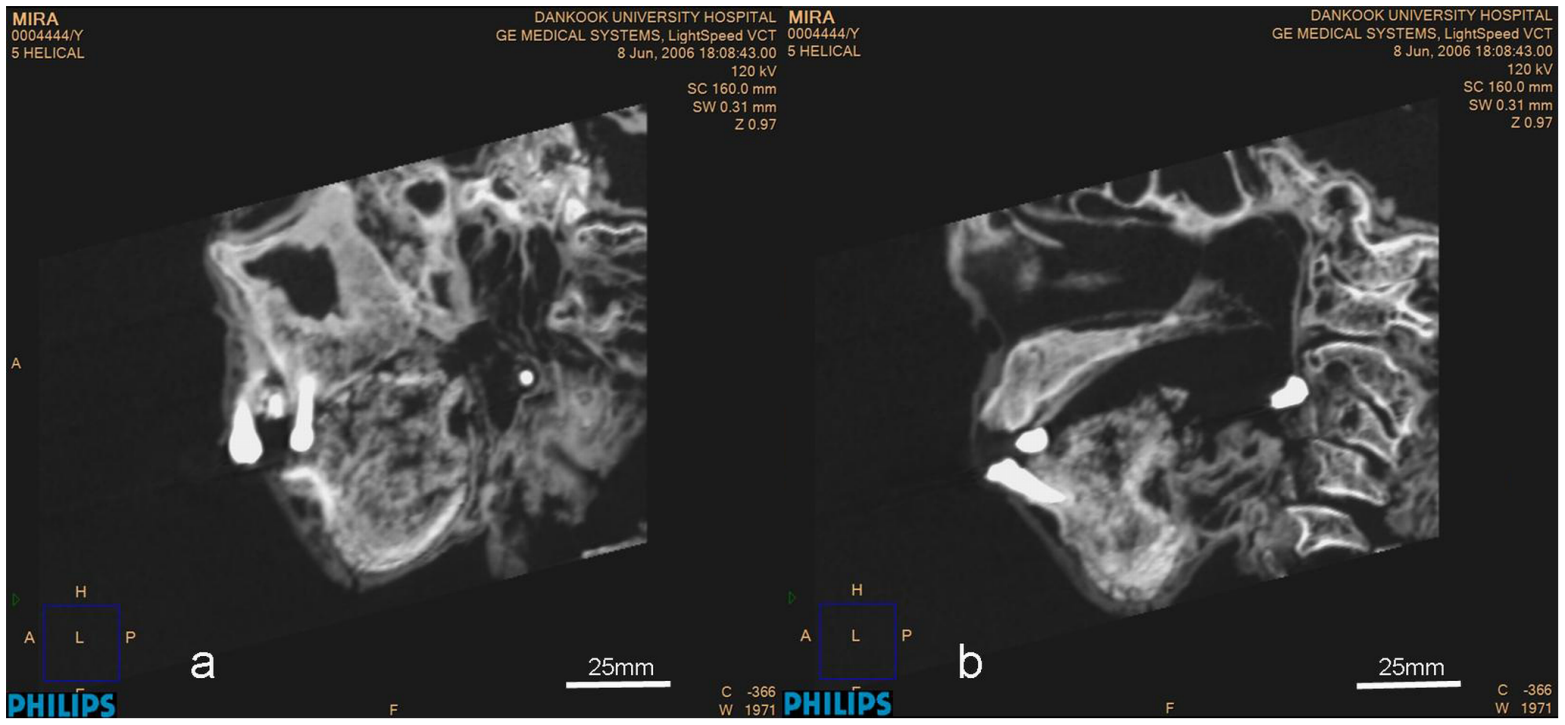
Sagittal (a) and parasagittal (b) CT sections through the lower face. The images demonstrate thickening of the maxillary walls and orbital roof, near-disappearance of cortical bone in the mandible, and resorption of dental roots.
